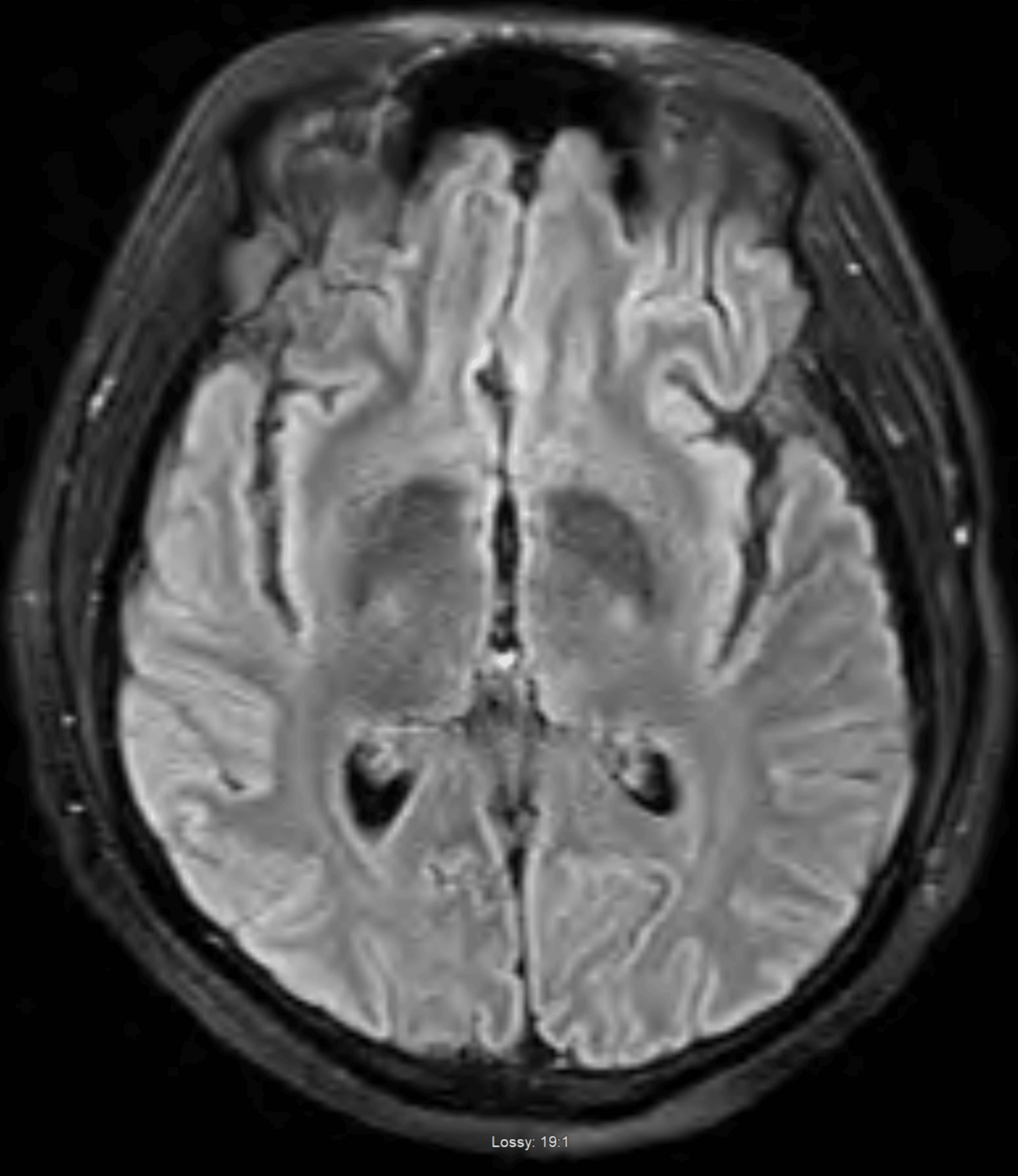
Axial MRI brain with and without contrast (T2 FLAIR sequence) showing no evidence of acute mass effect or cerebral edema. No space-occupying lesion is identified. FLAIR: fluid-attenuated inversion recovery
